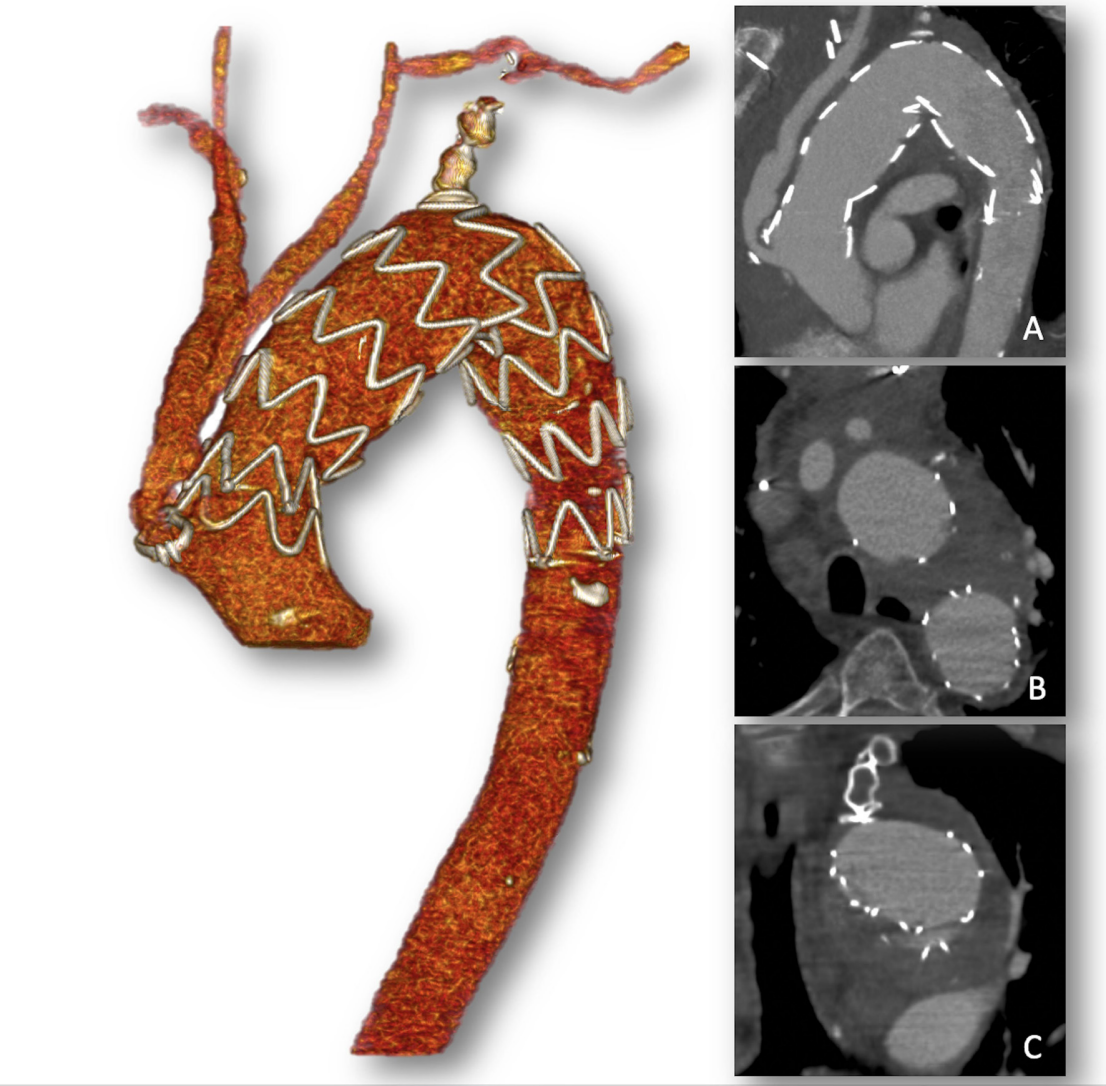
6-month follow-up computed tomography angiography 3D reconstruction showing complete exclusion of the aneurysm without any signs of endoleak, patency of the supra-aortic vessels, and no further aneurysm formation in the remnant aorta. (A) Sagittal view; (B) axial view; (C) coronal view.
